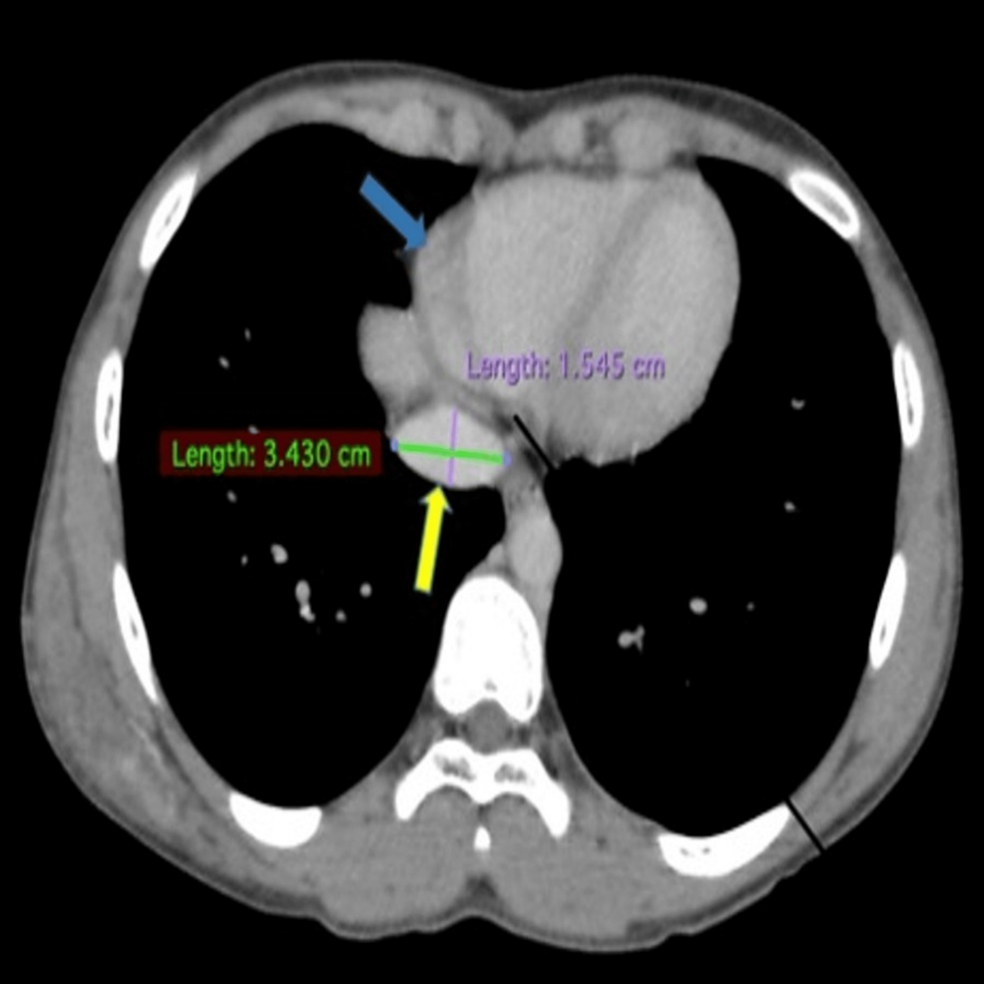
Axial CT scan image showing the inferior vena cava (yellow arrow) measurement taken at the level 2 cm distal to its insertion in the right atrium (blue arrow)
